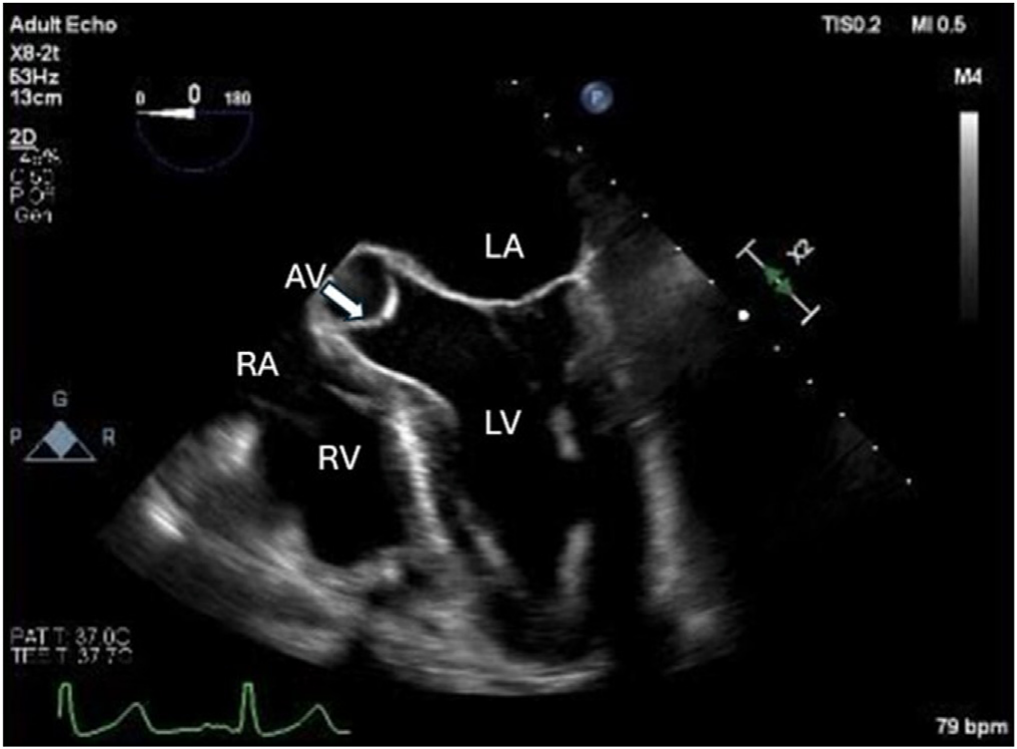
Transesophageal echocardiogramimage obtained during surgery. The 4 chambers of the heart are labeled along with the aortic valve. AV, aortic valve; LA, left atrium; LV, left ventricle; RA, right atrium; RV, right ventricle.
